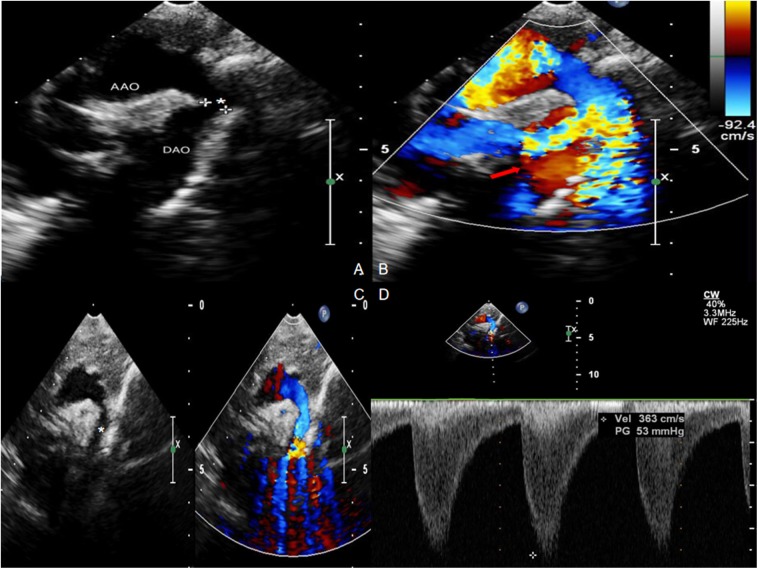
Echocardiogram with Color Doppler Flow Imaging showing coarctation of the aorta and increased blood flow velocity. (A,C) Coarctation of the aorta (asterisk). (B,D) Increased blood flow velocity (red arrow) with multicolored flow signal.
